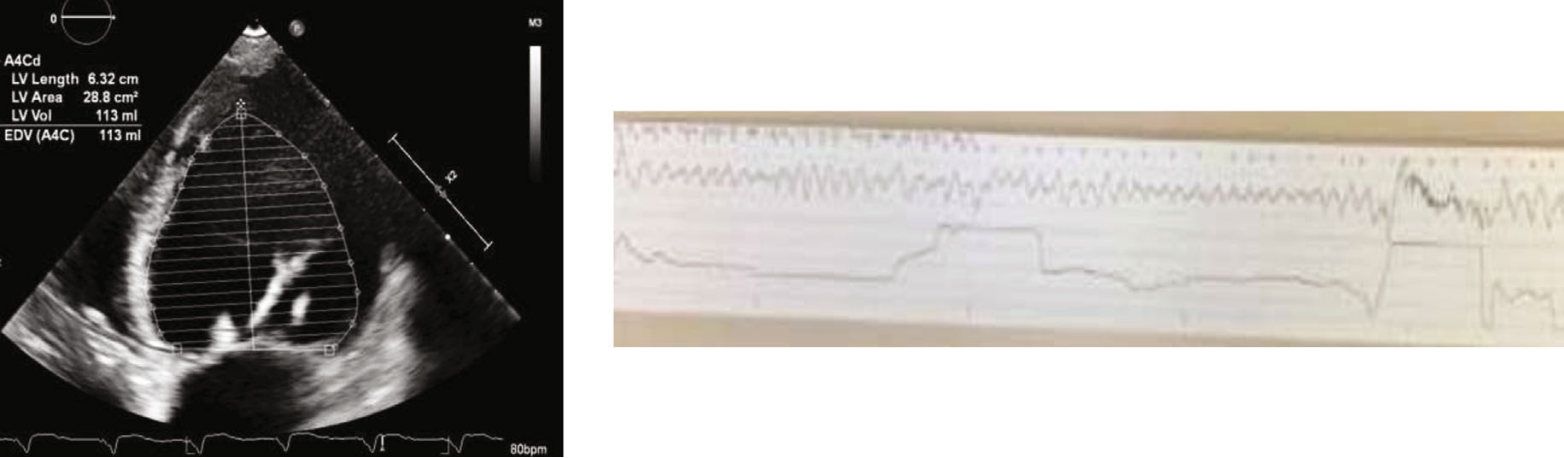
Case 1. Apical four-chamber view of left ventricle with Simpson disk summation method showing increased left ventricular end diastolic volume and diameter. Telemetry strip showing polymorphic ventricular fibrillation rhythm.
